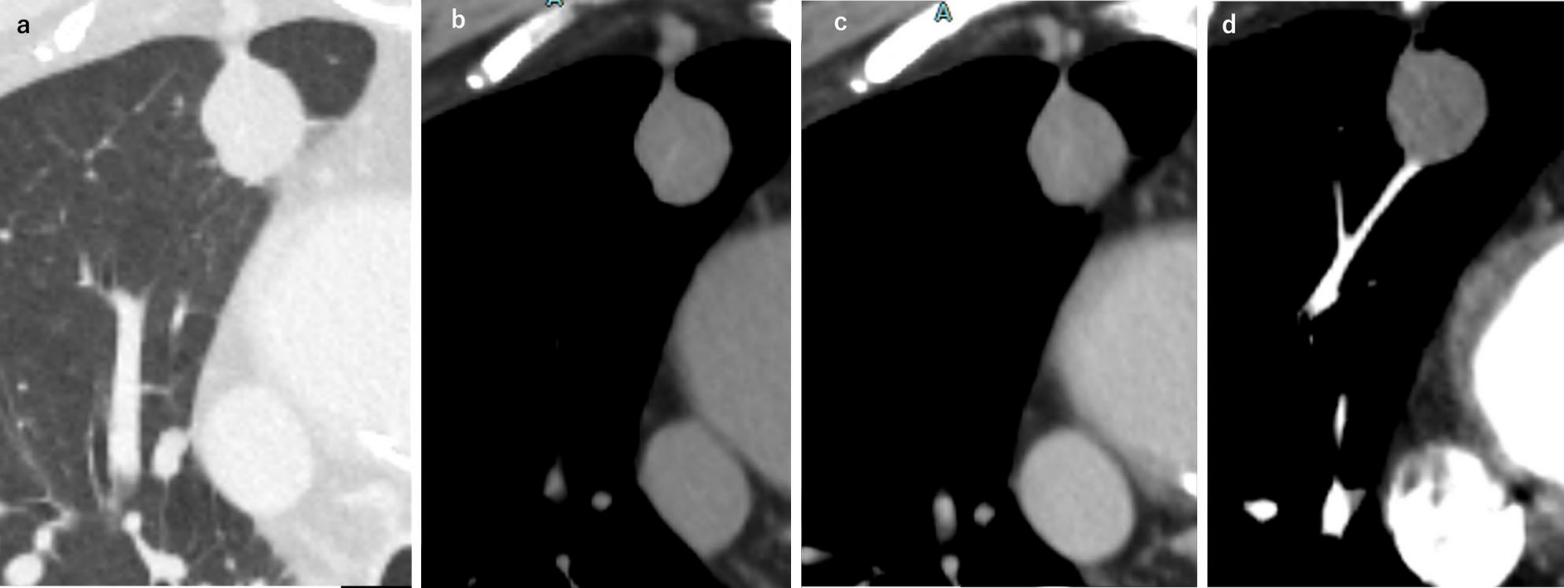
Parasitic embolism—pulmonary dirofilariasis. A man in his 70 s was diagnosed with pulmonary dirofilariasis. A solid nodule with well-defined margins was identified in the peripheral region of the right upper lobe (a). Non-contrast CT demonstrated faint internal calcification (b), and contrast-enhanced imaging showed no enhancement (c). The pulmonary artery adjacent to the lesion was continuous (d)
